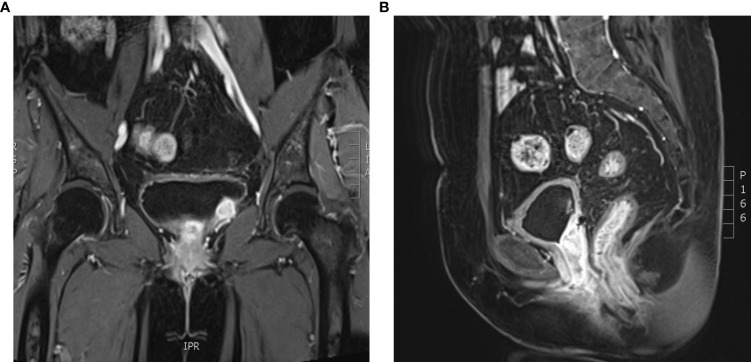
(A, B) Pelvic magnetic resonance imaging (September 23, 2020).
